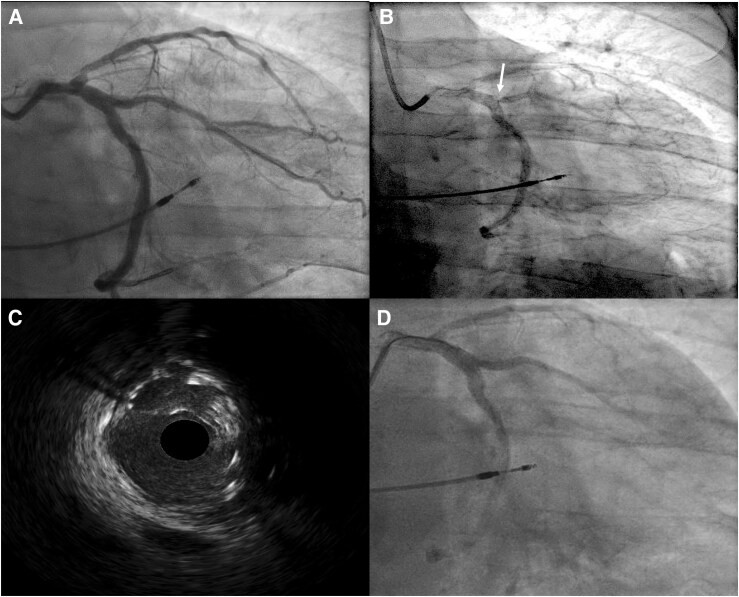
Coronary angiogram post-index PCI (*A*), the SST identified via coronary angiography (*B*) and intravascular ultrasound (*C*) and following angioplasty of the SST (D).
